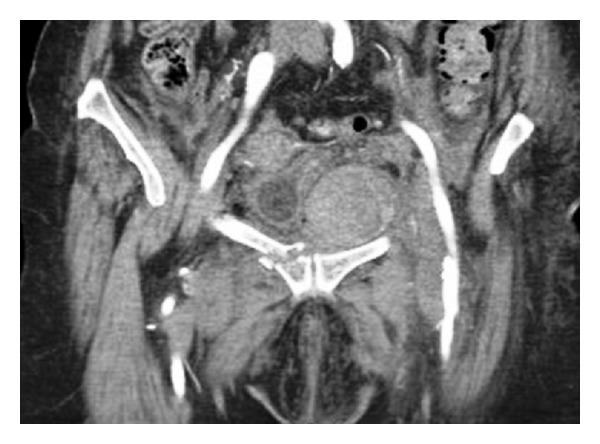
Coronal CT of the pelvis showing left haematoma neighboring the right fractured iliopubic ramus.
